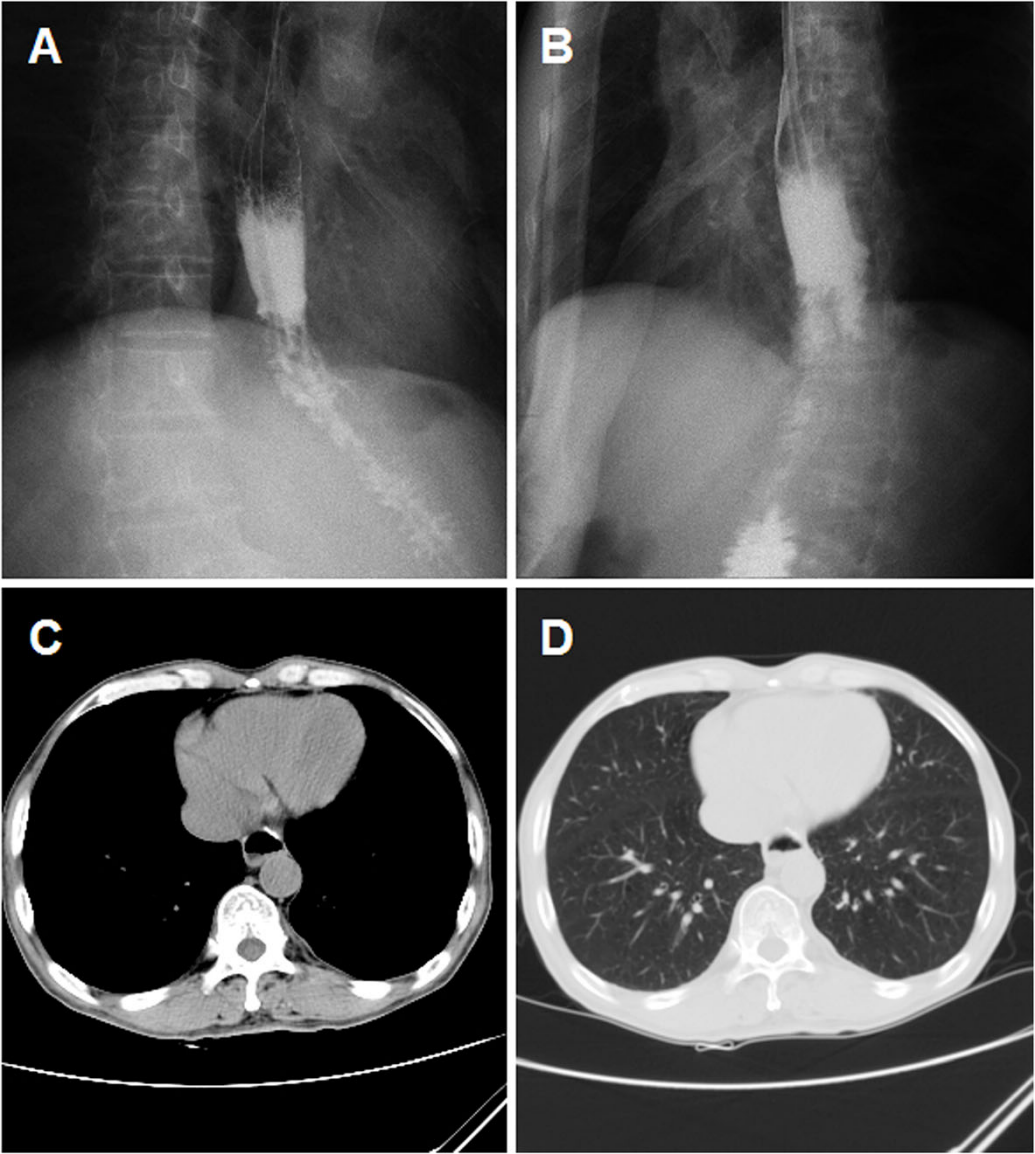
Examination after stent removal for case 24. a-b The drainage tube and stent were removed after procedure; esophagography shows that the contrast agent flows though the esophagus without any leakage. c–d The chest CT scan shows disappearance of mediastinal abscess, full expansion of the lungs with no pleural effusion
